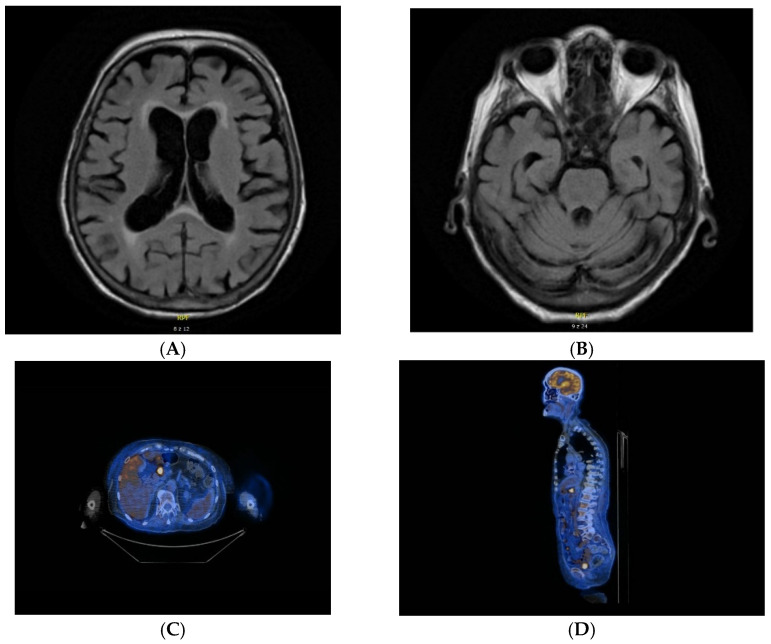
MRI and PET/CT results of a patient with cerebellar degeneration. (A,B) nonspecific vascular demyelination detected on MRI. (C,D) metabolically active area in the pylorus and in the locoregional lymph nodes detected on [18F]FDG PET/CT.
